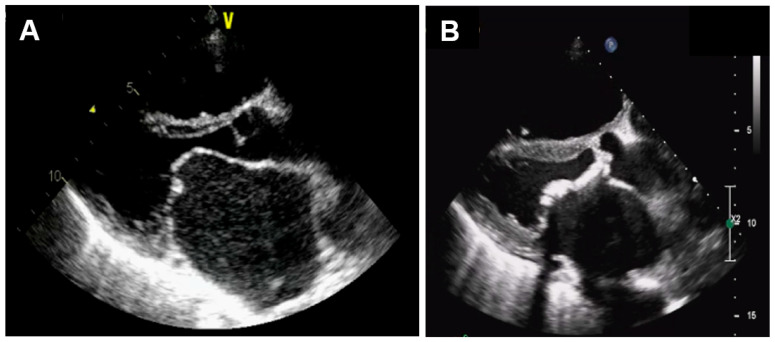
Echocardiographic findings of mitral valve in rheumatic mitral stenosis. (A) Parasternal long-axis view of patient in earlier stage, showing normal thickness in valve with minimal calcification. (B) Parasternal long-axis view of different patient with concomitant rheumatic aortic stenosis presenting in later stage, showing extensive calcification of anterior mitral leaflet and aortic valve.
